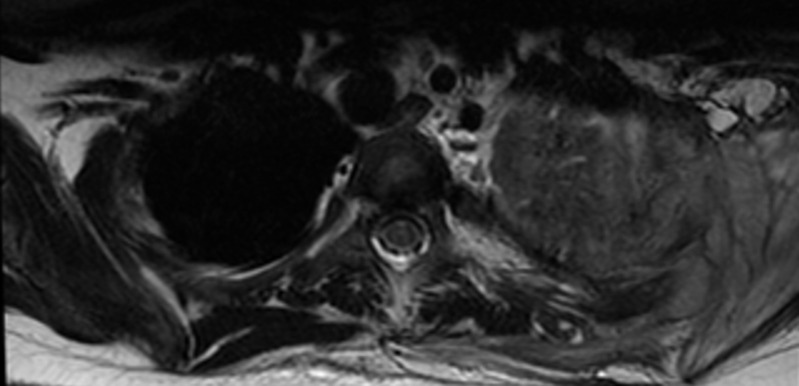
magnetic resonance imaging showed destructive left apical /supraclavicular enhancing lesion, which invades the peripheral brachial plexopathy
